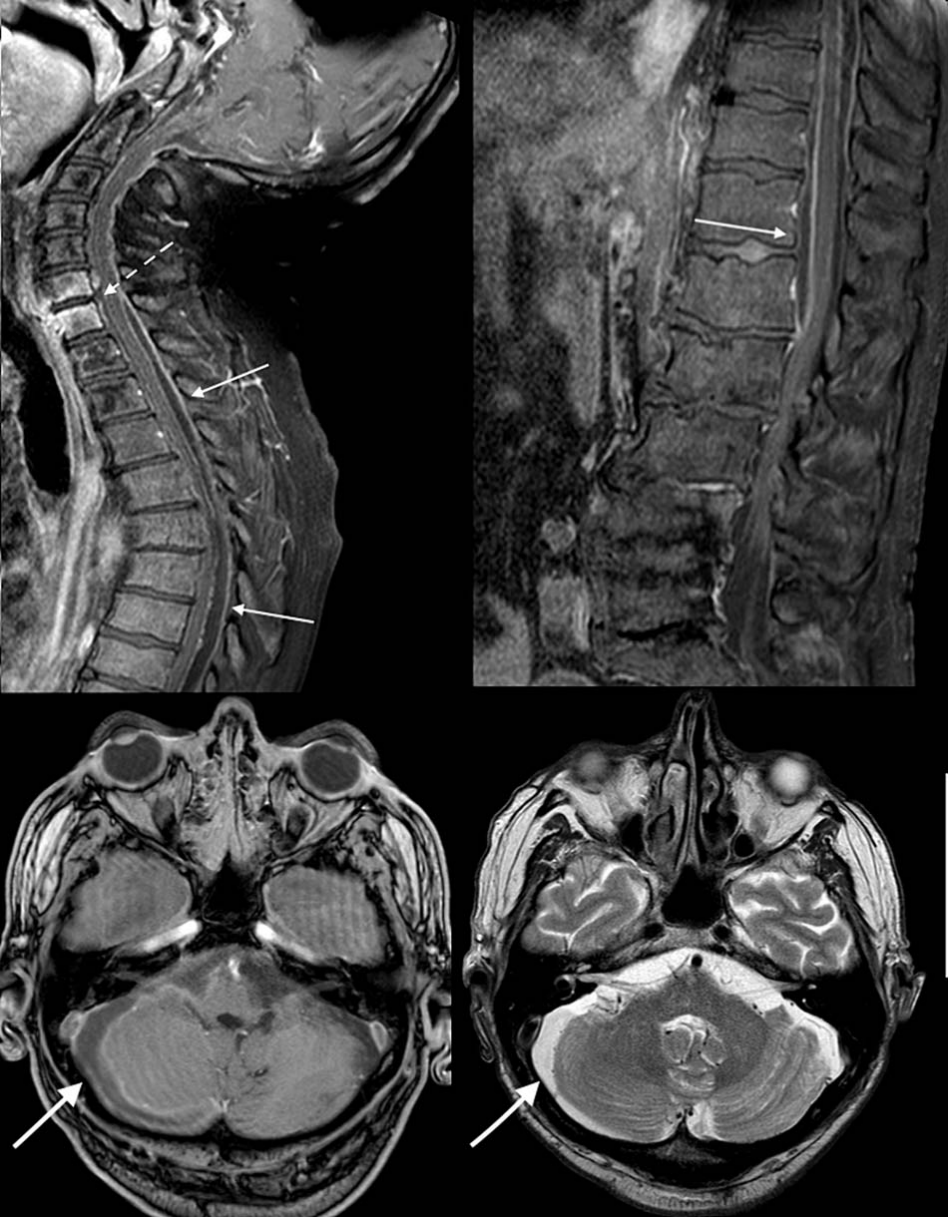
Initial MRI brain and whole spine demonstrating vertebral C6-C7 osteomyelitis (dotted arrow), subdural abscess within the cerebellum (bold arrow), and an epidural abscess within the spinal canal (arrow).
